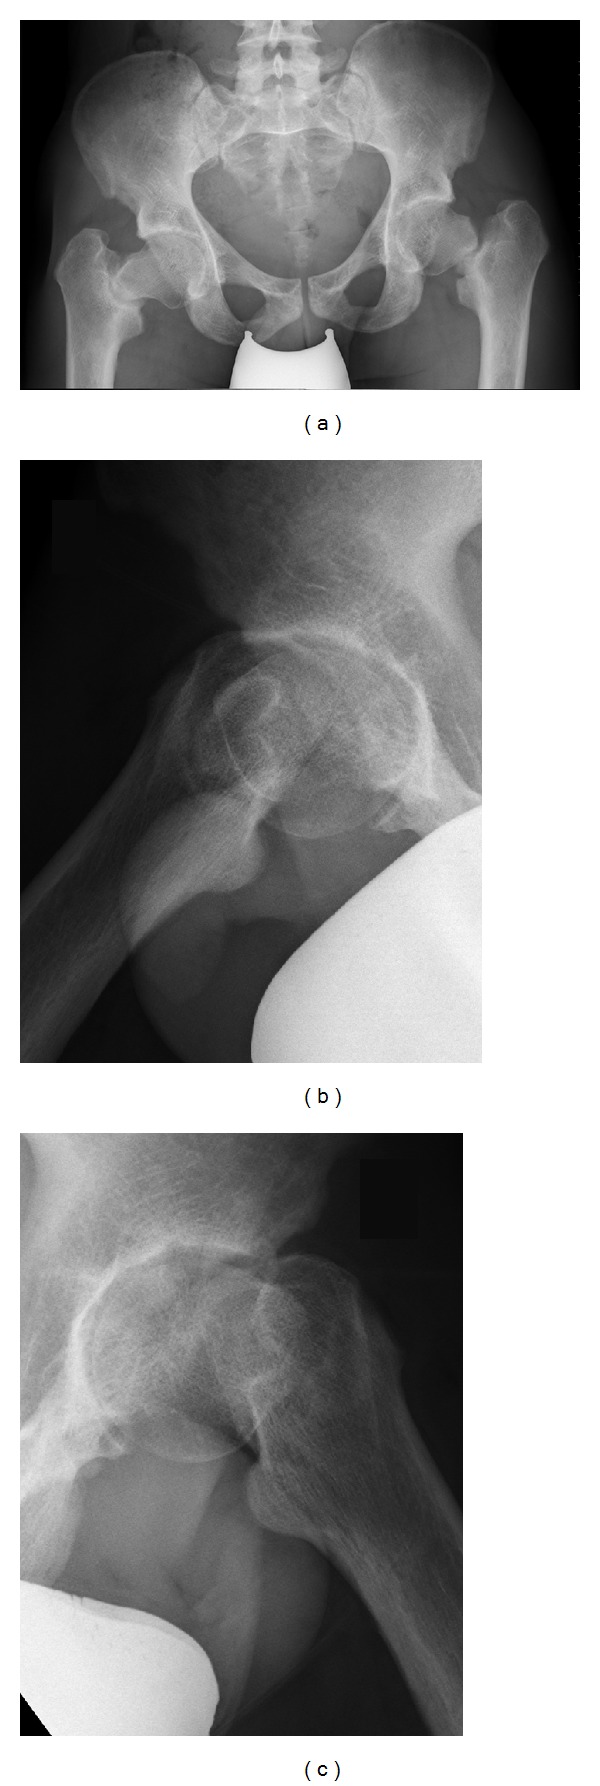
Standard pelvic radiograph showing bilateral pseudarthrosis of the femoral neck (a), axial radiograph of the right hip (b), and axial radiograph of the left hip (c).
